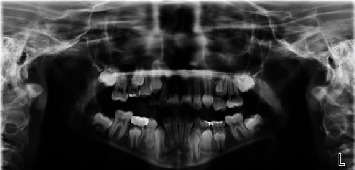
Initial screening panoramic radiograph.
